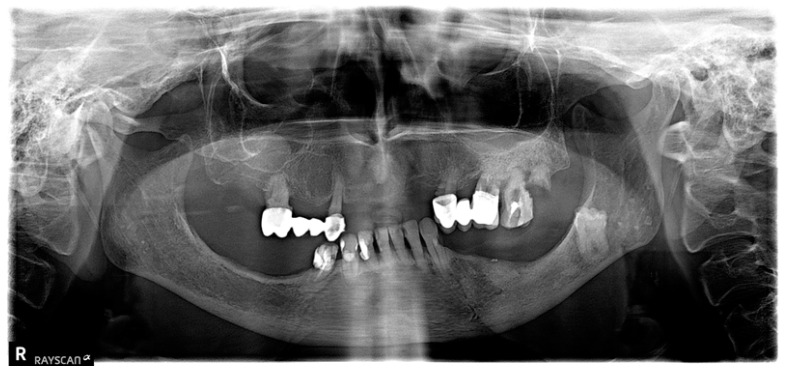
X-ray of the same patient as in Figure 5 after completion of treatment. Visible complete lack of necrotic changes in the mandibular bone.
